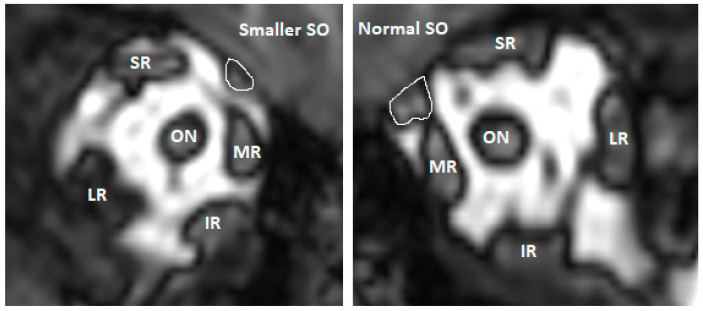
Example of a smaller right superior oblique (SO) muscle in one of the patients with non-syndromic UCS on MRI coronal plane. Abbreviations: SR: superior rectus, MR: medial rectus, LR: lateral rectus, IR: inferior rectus, ON; optic nerve.
